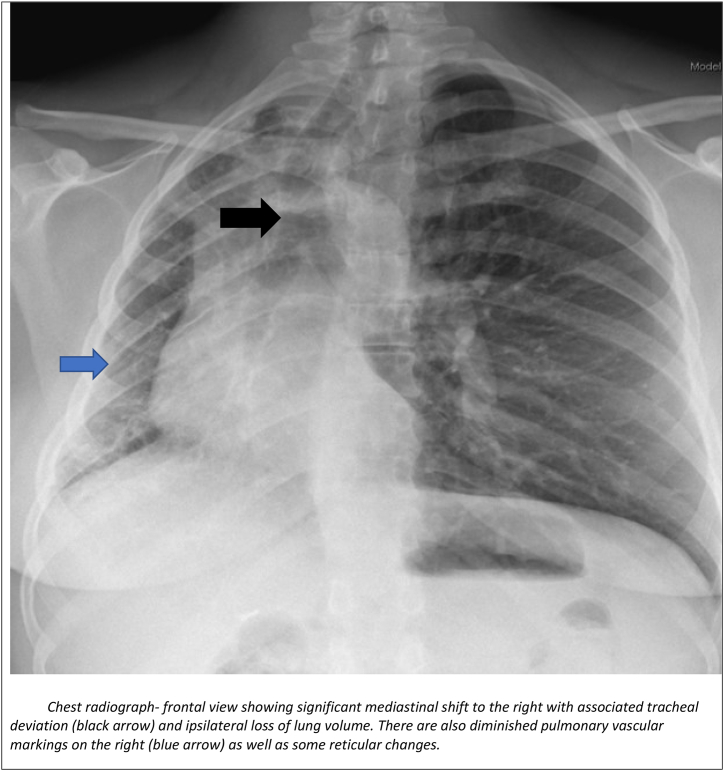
Chest radiograph-frontal view showing significant mediastinal shift to the right with associated tracheal deviation (black arrow) and ipsilateral loss of lung volume. There are also diminished pulmonary vascular markings on the right (blue arrow) as well as some reticular changes.
